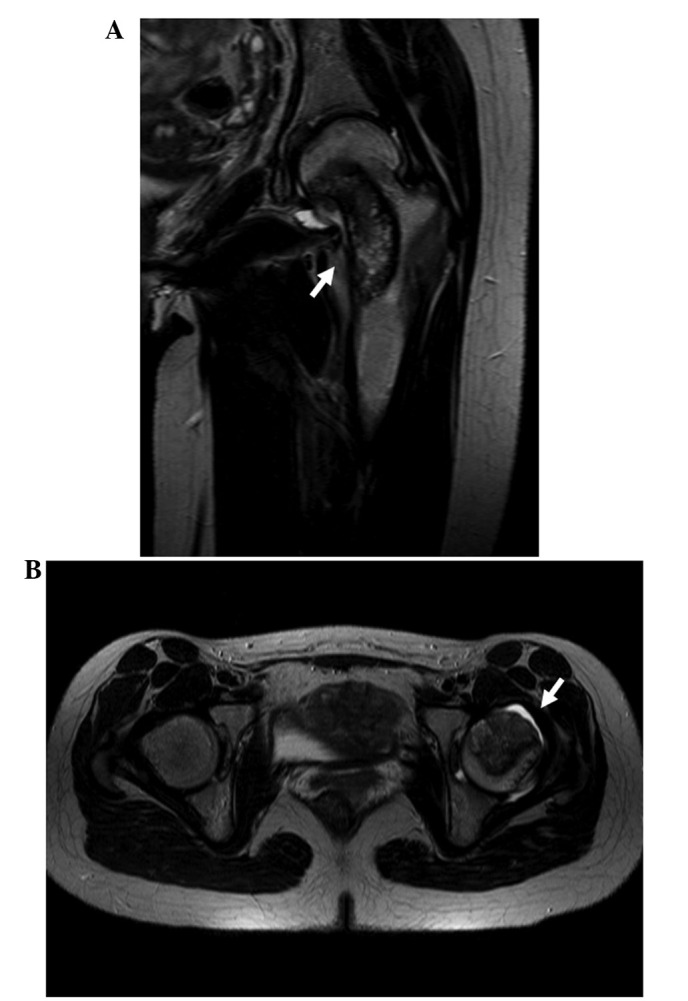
Magnetic resonance imaging (MRI) observations. (A) T2-weighted coronal imaging and (B) T2-weighted axial imaging. MRI showing a heterogeneous tumor arising from the femoral neck and extending to the trochanteric site, which is hypointense compared with the bone (arrow). In the capsule of the hip joint, a high intensity area is apparent (arrow).
